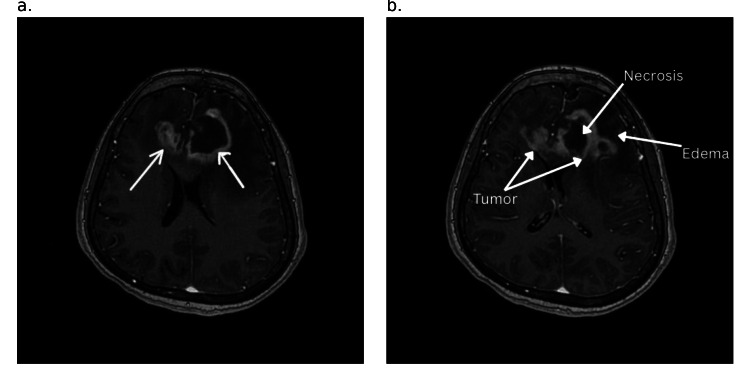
Axial contrast-enhanced MRI image The image shows a peripherally enhancing tumor (shown in white arrow in figure 1a) in the left anterior frontal lobe extending into the right frontal lobe through the genu of the corpus callosum: a. At the level of the body of the lateral ventricles. b. At the level of the capsuloganglionic region MRI: magnetic resonance imaging
